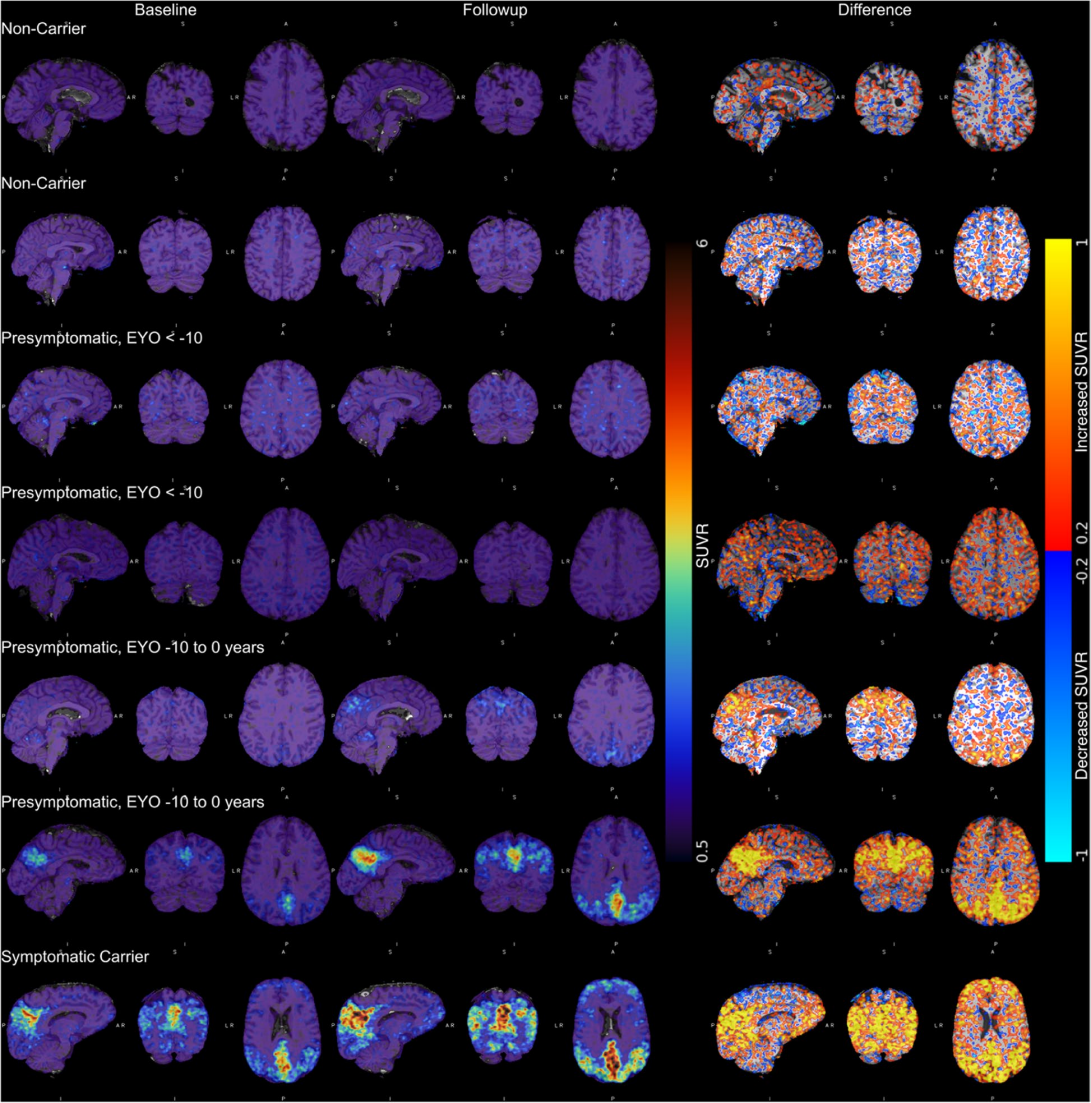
Longitudinal tau PET images from a selection of ADAD family members. The images have been smoothed with a Gaussian kernel (full-width at half maximum = 3 mm) in order to provide easier visual interpretation. The first two columns represent three cardinal slice views on the precuneus at baseline and follow-up time points. All of these SUVR images are mapped to the same colour scale. The third column represents the difference image between the two time points, with warm colours showing an increase in tau deposition and cool colours representing a decrease
